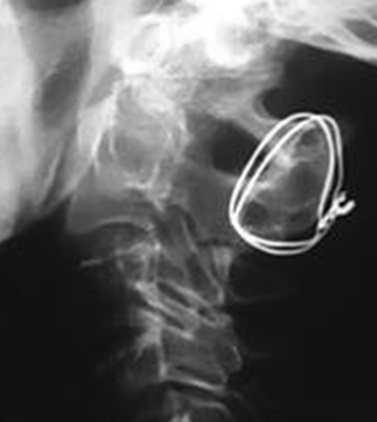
Post operative standard X-ray showing a patient who benefited from wiring
